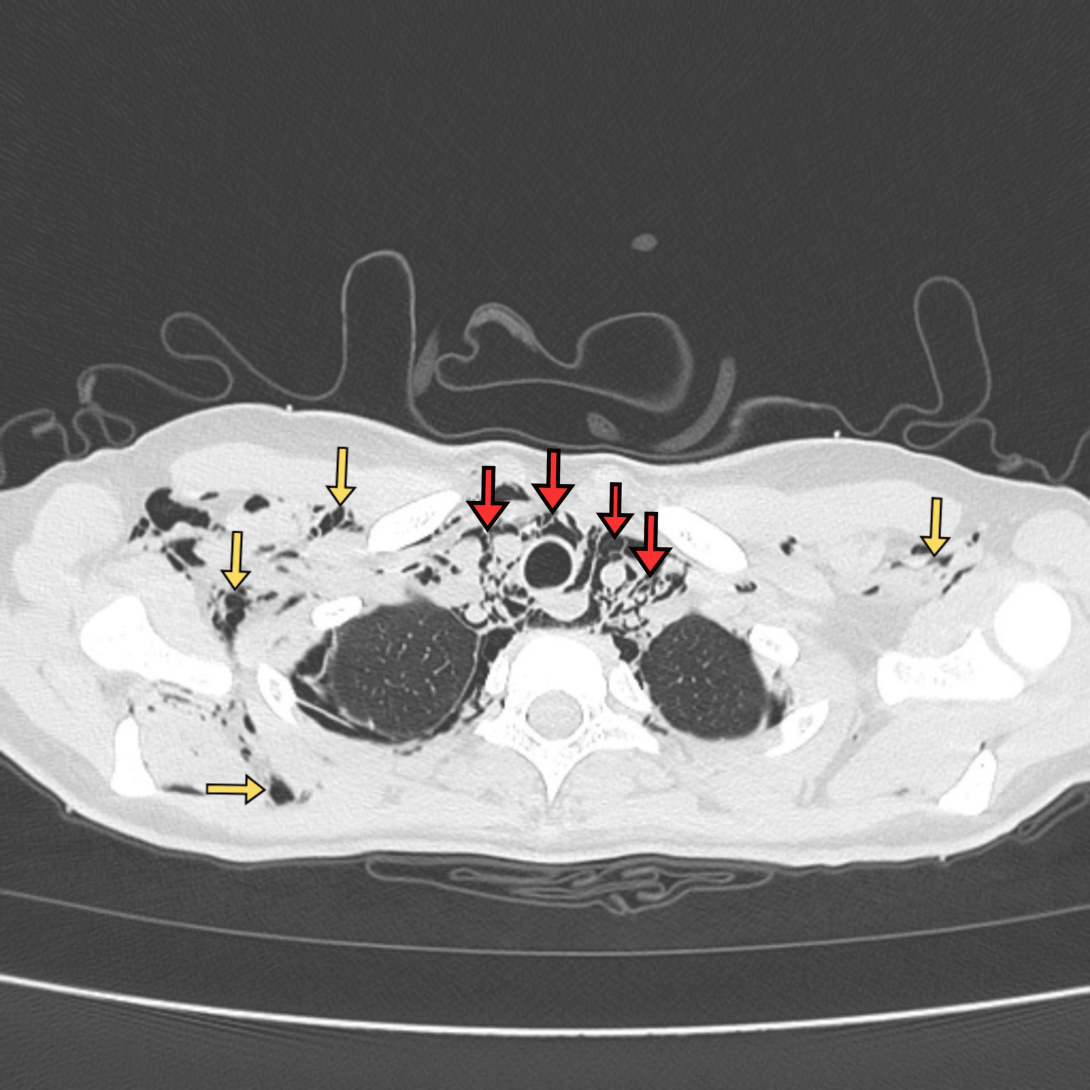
Axial CT chest Axial CT chest showing subcutaneous emphysema (yellow arrows indicate air pockets in the subcutaneous tissues of the neck and axilla) and pneumomediastinum (red arrows highlight mediastinal air around the paratracheal region)
